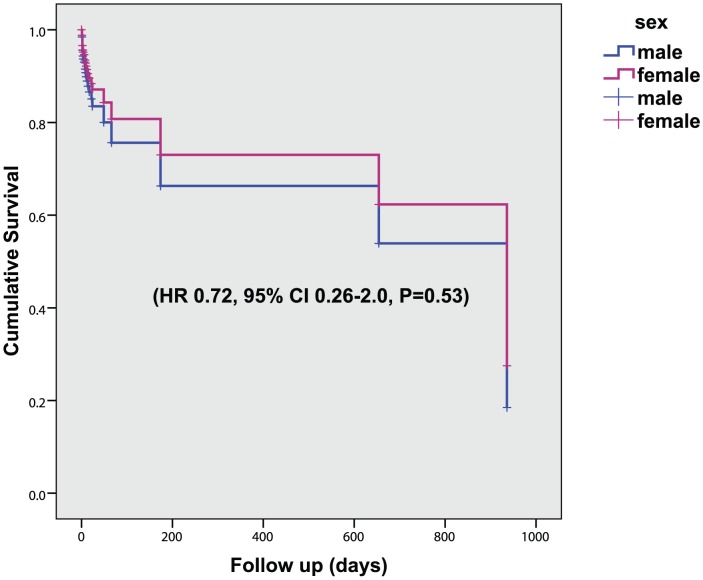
Cumulative survival of all recurrent cerebral ischemic events in men and women with MRI PH +ve high grade stenosis (70–99%).
